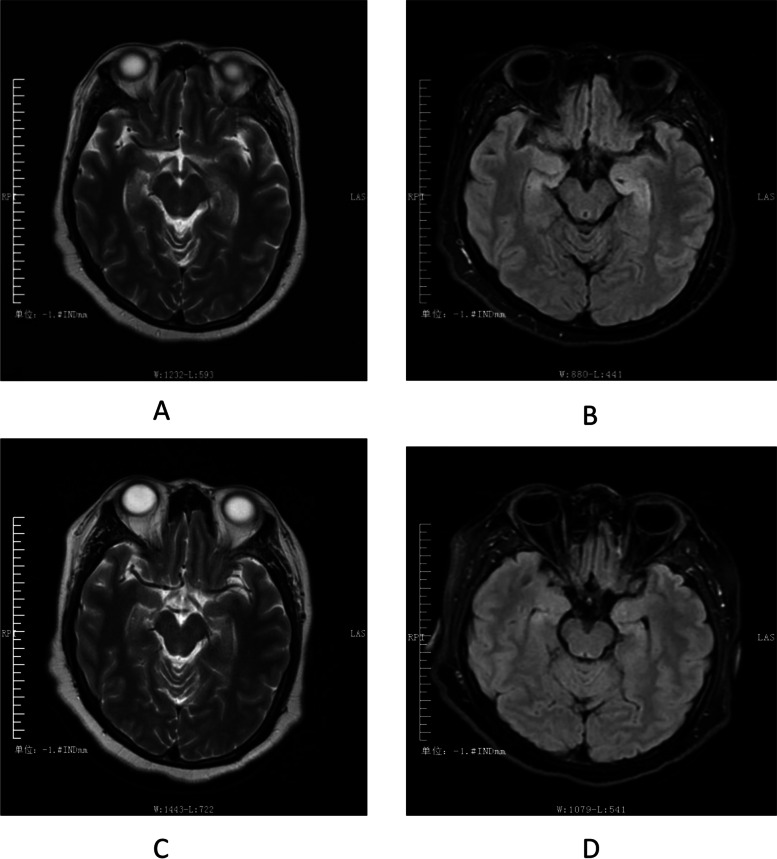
MRI image of the patient (Example 1). T2W1 showed a high signal (a), Flair showed a high signal (b), and the abnormal signal in the hippocampus disappeared after six months of immunotherapy (c, d)
